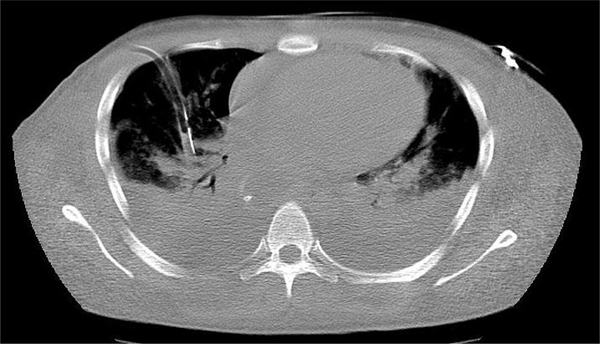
A IV contrast enhanced CT scan of the thorax, demonstrating bilateral severe pleural effusions and right pleural chest drain insertion.
